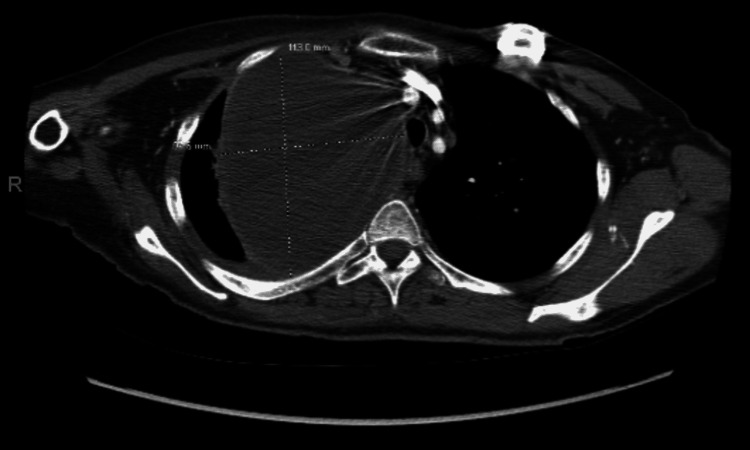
Chest CT showing a large, moderately enhancing mass in the right upper lobe.
